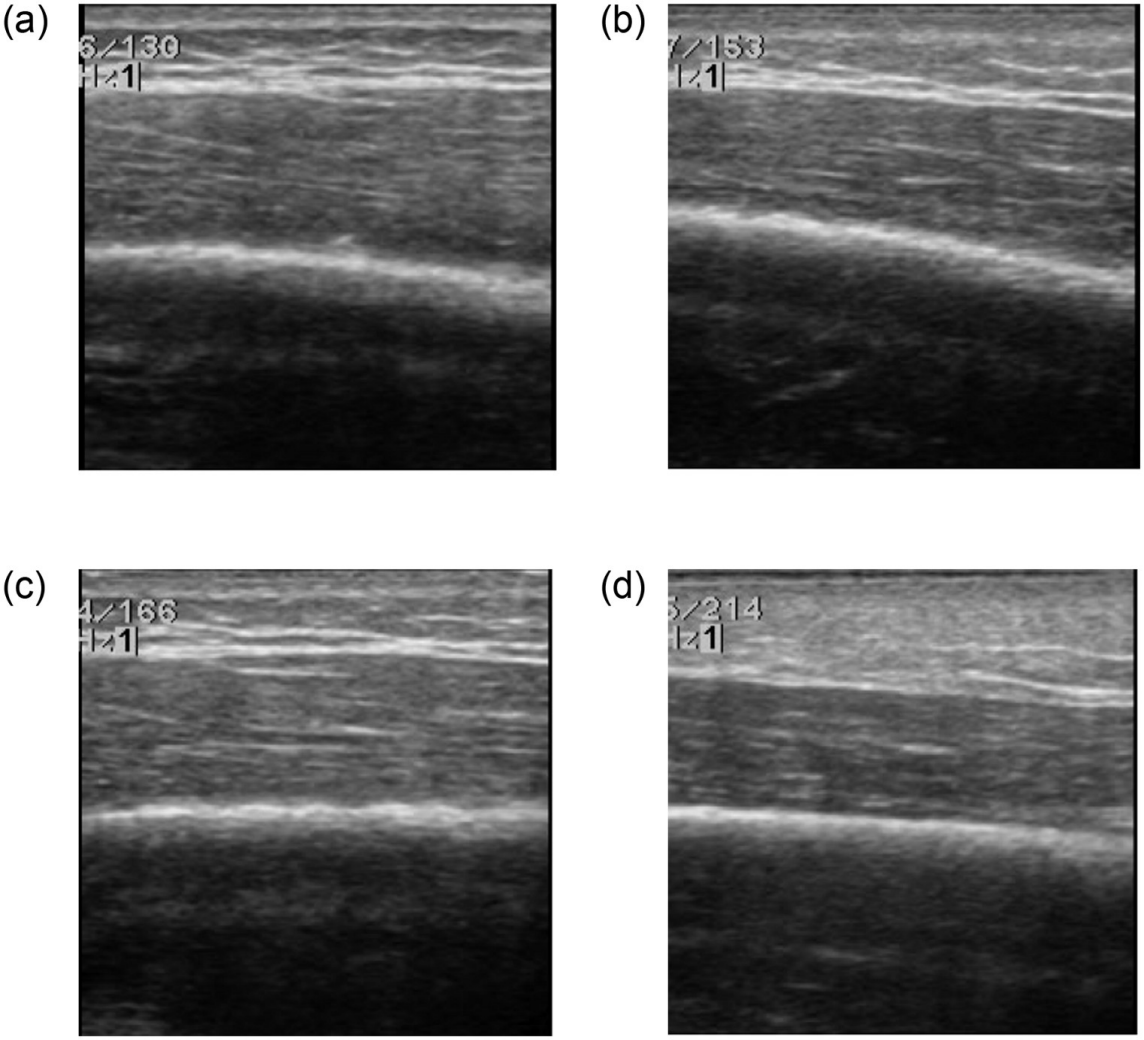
Typical examples of gray-scale ultrasound images before and after cupping therapy from a person. The changes between pre-cupping (a & c) and post-cupping (b & d) are not easily described by visual examinations. (a) Ultrasound image of the triceps of the dominant side before applying cupping therapy at −225 mmHg for 5 min. (b) Post-cupping ultrasound image from the condition of (a). (c) Ultrasound image of the triceps of the dominant side before applying cupping therapy at −225 mmHg for 10 min. (d) Post-cupping ultrasound image from the condition of (c).
